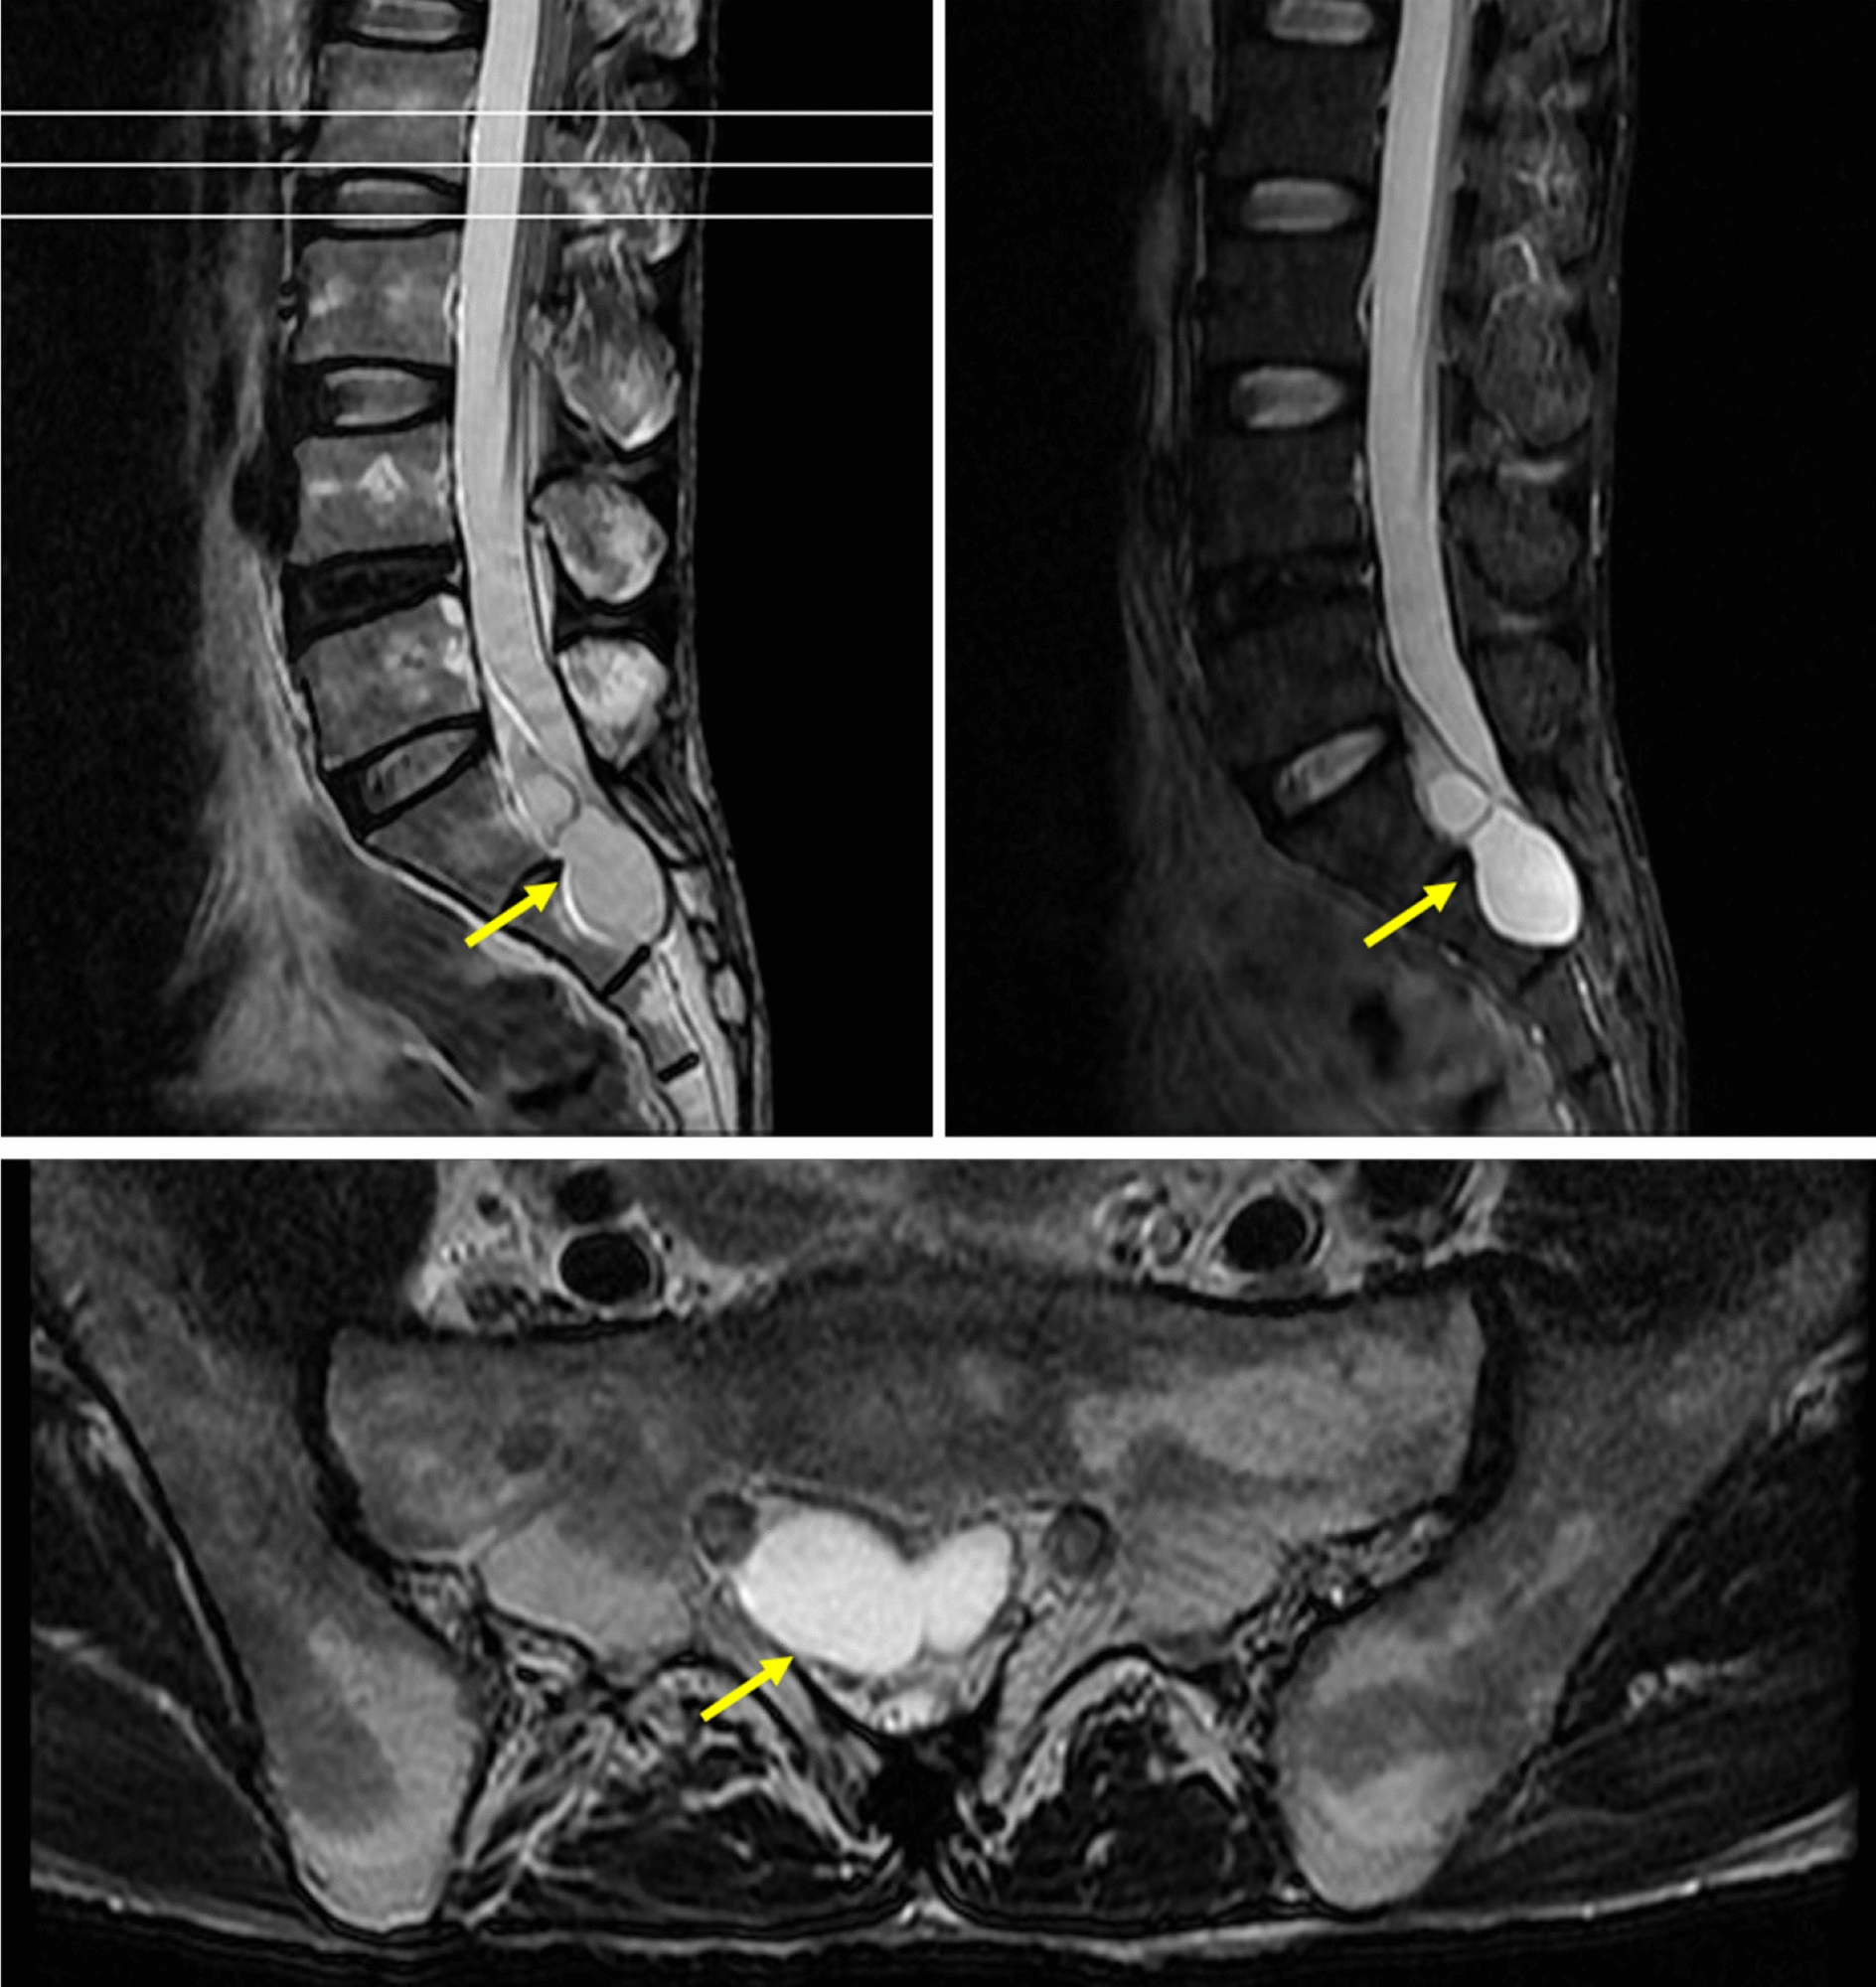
Preoperative lumbar spine MRI. T2 signal shadows in the S1–2 spinal canal
